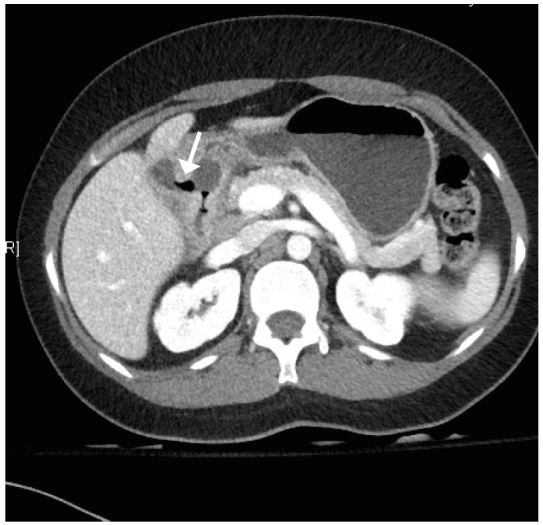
Transverse computed tomography of air passing from duodenum through a fistula into the gallbladder.
